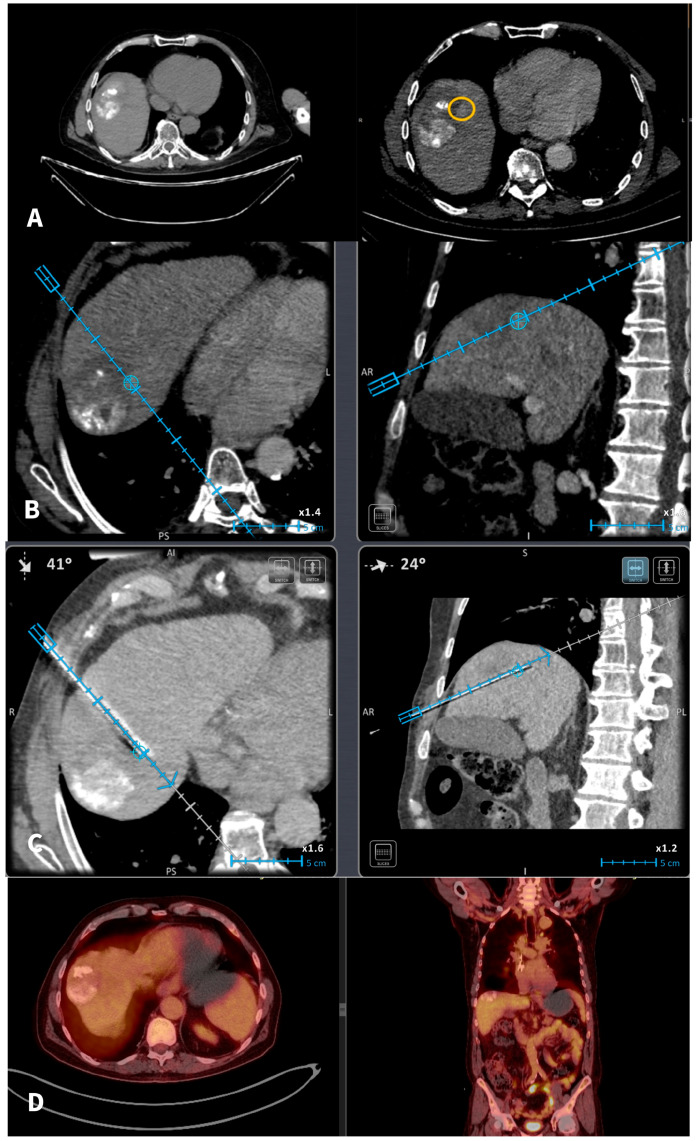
A 67-y-o male HCC patient who underwent in 2020 a combined therapy with MWA and transarterial chemoembolization. (A): In 2022 imaging follow-up depicts solitary hepatic lesion located in the hepatic segment VIII, 1 cm in diameter (recurrent HCC). The recurrent tumor was non-visible in the NCMCT scan. Axial CT scans pre and post-intravenous injection of Iodine contrast (portal venous phases) depicting the recurrent tumor (yellow circle) anteriorly and medially to the previously TACE + MWA zone of necrosis. (B): A commercially available electromagnetic navigation system with a marker software (blue circle) is used for tumor targeting and navigation of the microwave probe. (C): A commercially available electromagnetic navigation system with a marker software (blue circle) is used for navigation of the microwave probe. (D): Follow-Up PET-CT scan 6 months after treatment verifies total tumor necrosis.
